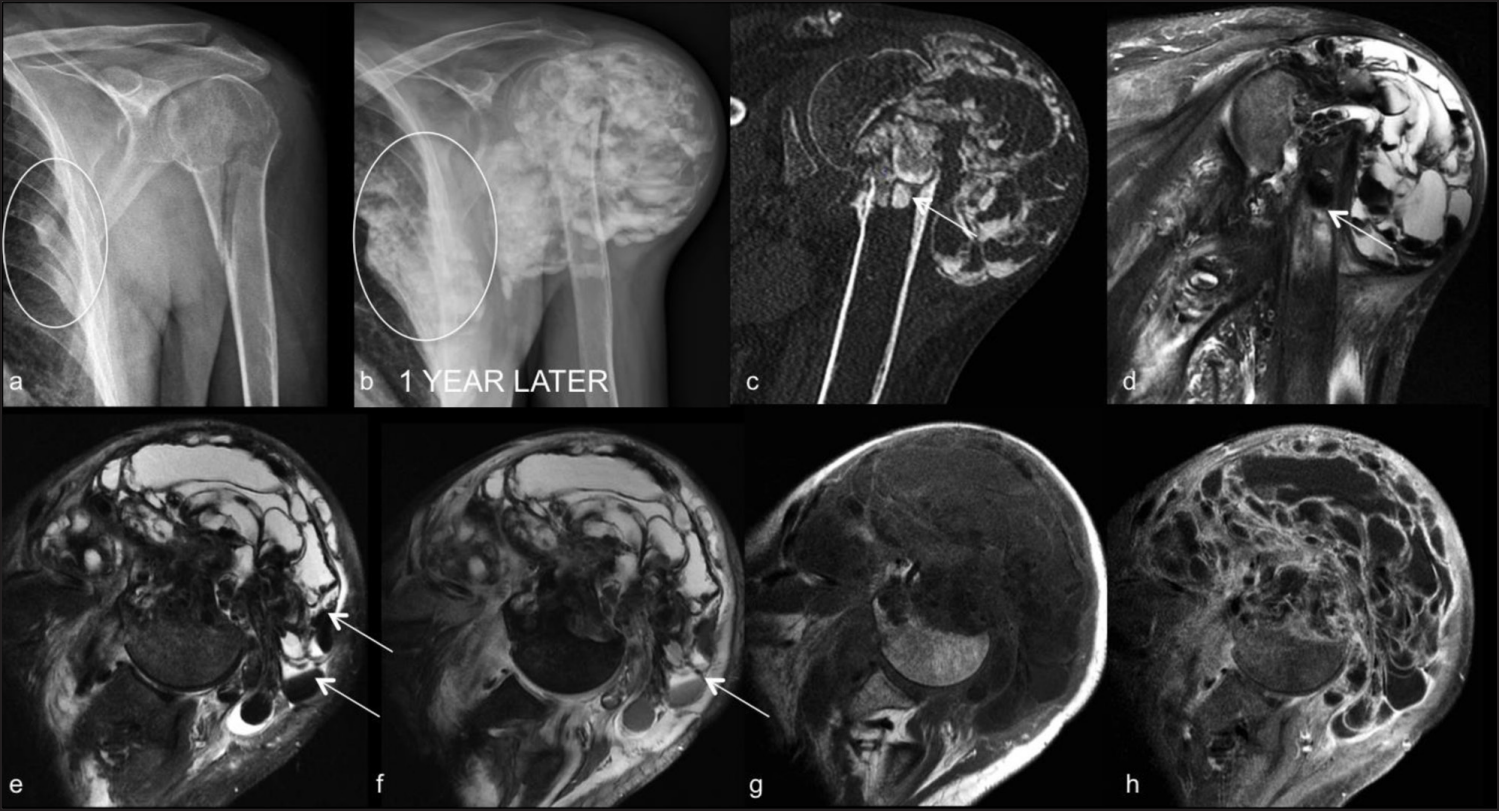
Plain film shows a proximal humeral fracture and rib fractures (circle in 1a). Plain film 1-year later shows a giant mass involving the left shoulder and a smaller one on the costal grill (circle), both centred on the previous fracture sites (1b). CT exam, coronal reconstruction, reveals multiple calcifications within the mass and an extension into the humeral diaphysis (arrow in 1c). MRI exam (coronal T2-WI Fat Sat in 1d; axial T2-WI Fat Sat in 1e; axial GRE sequence in 1f; axial T1-WI pre- and post- gadolinium administration in 1g, h) shows the proximal humeral endomedular extension (arrow in 1d) and depicts multiple fluid-calcium levels within the cystic spaces of the lesion (sedimentation sign, arrows in 1d–f), better demonstrating the heterogeneity and the extension of the mass. Contrast-enhanced T1-WI (1h) shows thin peripheral enhancement of the cystic spaces and rules out solid or suspicious components. Abbreviations: CT: computed tomography, MRI: magnetic resonance imaging, T1- and T2-WI: T1- and T2-weighted imaging, GRE: gradient recalled echo.
